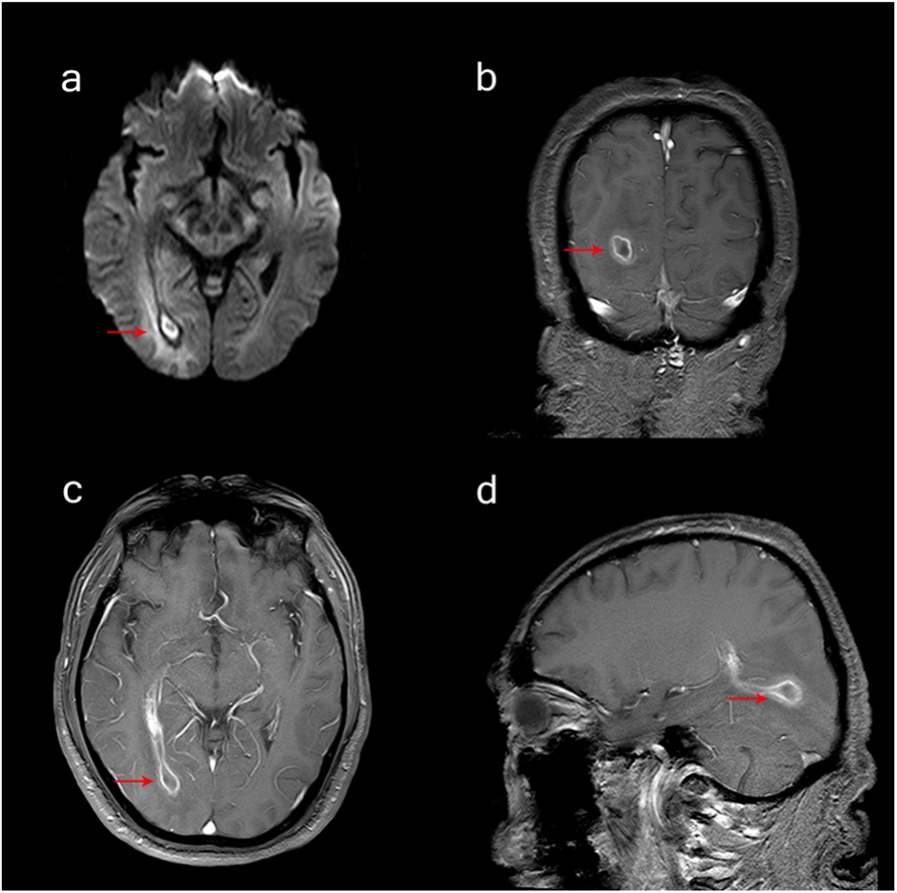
Follow-up brain MRI findings. After 2 weeks Penicillin G treatment, the size of the abscess was significantly reduced: (a) DWI and (b-d) gadolinium enhanced
